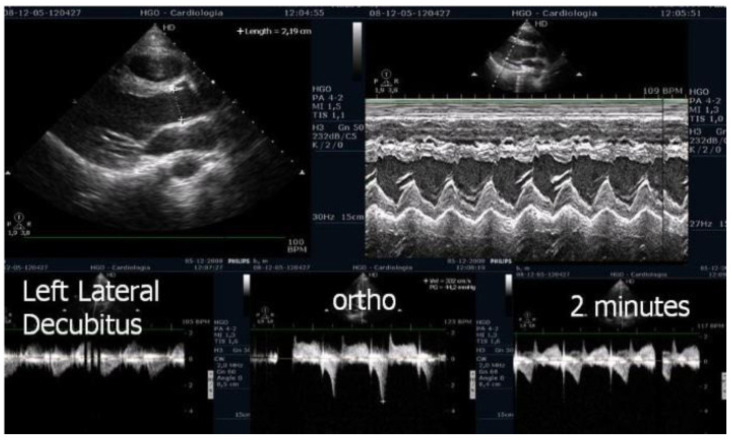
Echocardiography before exercise in a symptomatic adolescent in the left lateral decubitus position, and in the orthostatic position before and at the beginning of exercise [3].
